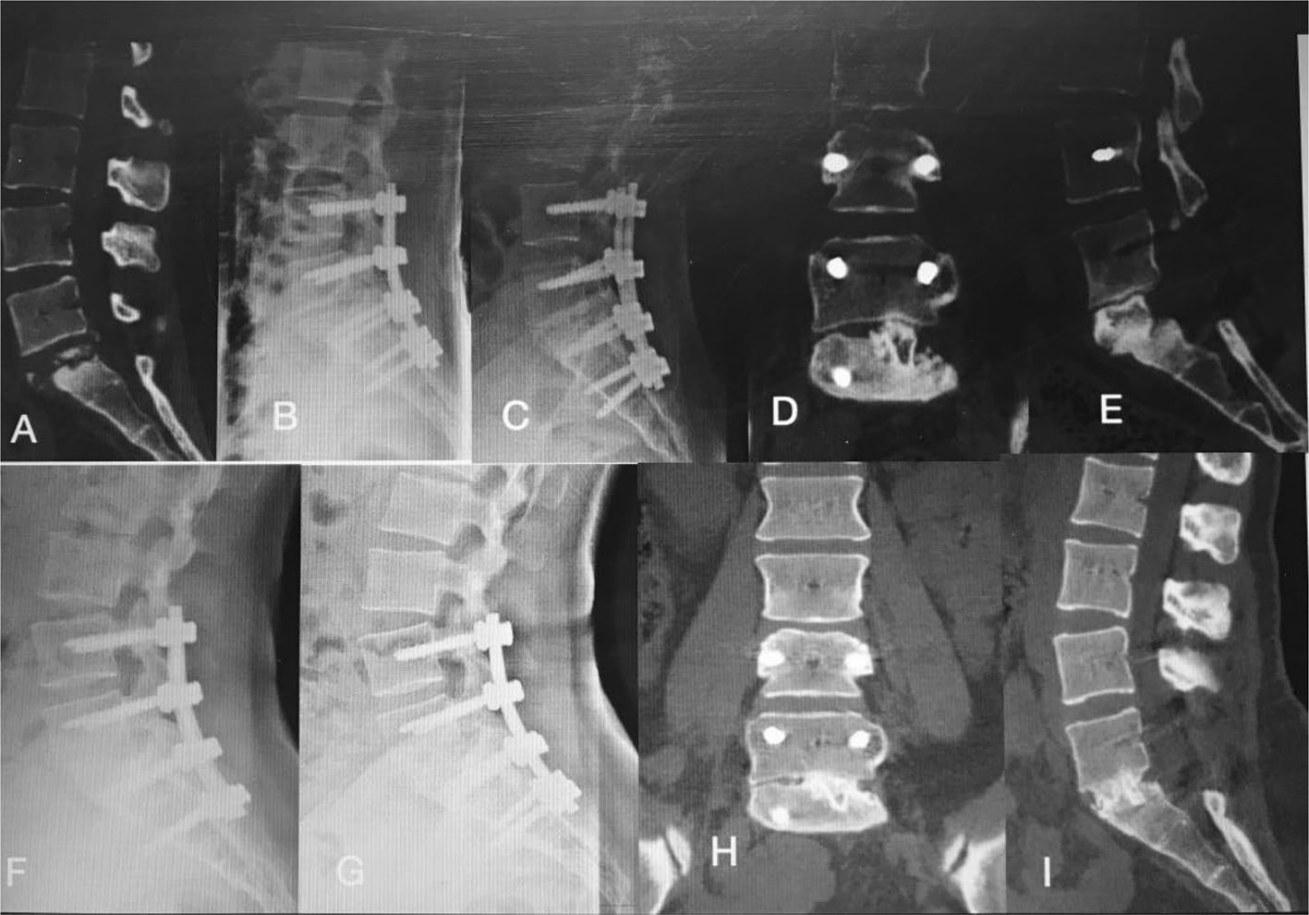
A 28-year-old female patient with lumbosacral spinal tuberculosis (L5-S1) underwent posterior debridement and decompression combined with instrumentation. (A,B) Preoperative computed tomography (CT) shows bone destruction of the L5-S1 vertebrae and compression of the nerve, and 1-week X-ray shows the correction was maintained. (C–E) The 6-month postoperative X-ray and 6-month postoperative CT show the correction was maintained, but the bone was not fully healed. (F) The 1-year and 2-year postoperative X-rays show the kyphosis correction has been maintained. (G–I) At 4-year follow-up, plain X-ray and CT show solid bone fusion.
